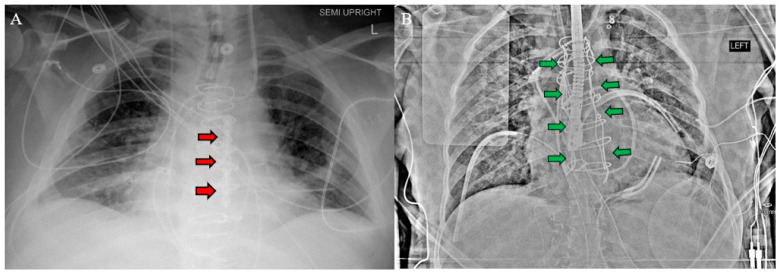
Postoperative chest X-ray for (A) Patient 1 and (B) Patient 2 demonstrate sternal plate used in Patient 1 (red arrows A) and Robicsek technique used in Patient 2 (green arrows B).
